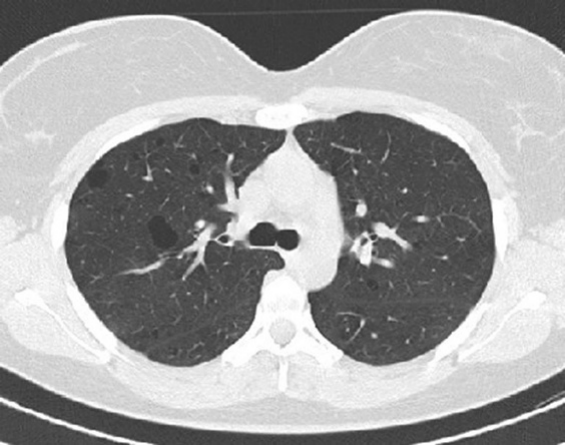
A 20-year-old woman, non-smoker, with PLCH; pulmonary cysts at her first computed tomography (CT) examination. The patient was treated in the neonatal period for a cutaneous form of Langerhans cell histiocytosis that regressed completely during infancy. The cysts are postulated to have resulted from granolomatous transformation of pulmonary histiocytosis, possibly concurrent with the cutaneous paediatric disease. Cysts remained stable during 10 years of follow-up
